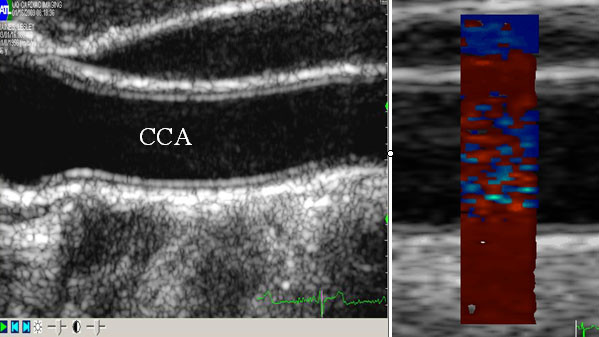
Carotid 2D and TDI imaging. Digitized longitudinal 2D scan of the common carotid artery (CCA) just prior to the bifurcation (right) and optimized ROI window with color tissue Doppler of the CCA.
